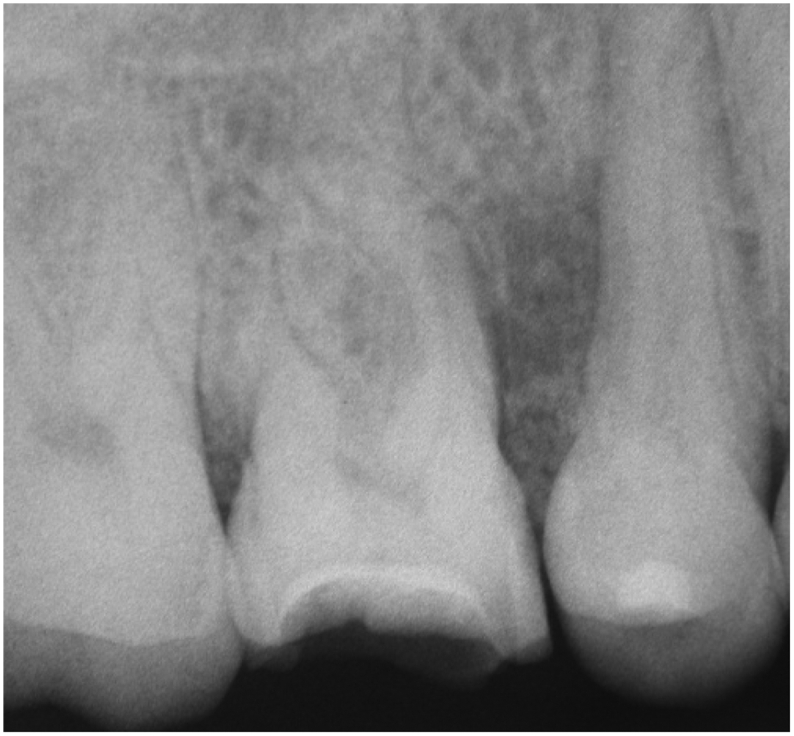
Pré-operative radiography showing the radiolucency between the first molar and second premolar.
